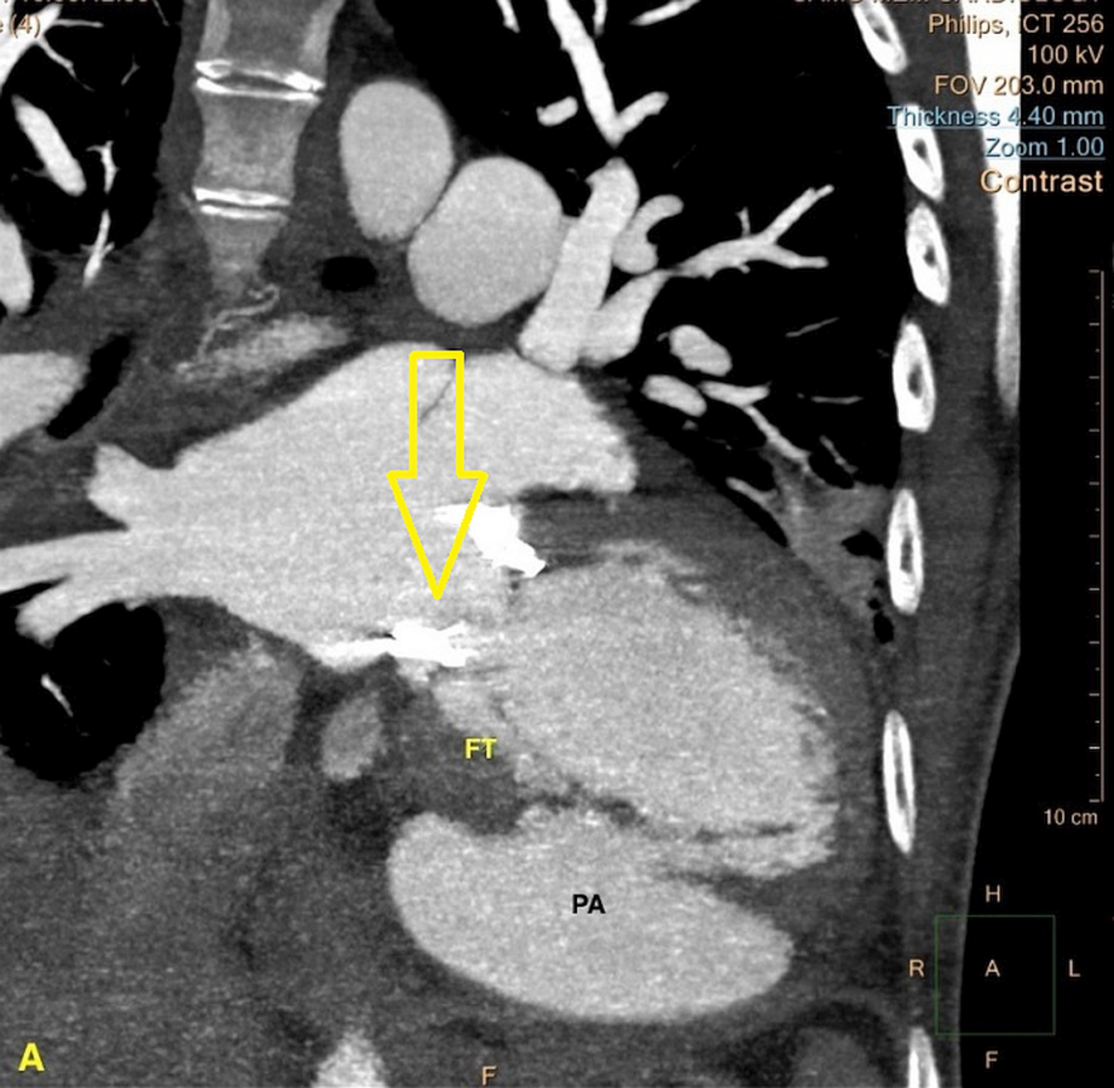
Cardiac computed tomography (cardiac CT) image showing the fistulous tract (FT) communicating from the mitral valve to the left ventricular pseudoaneurysm (PA).
